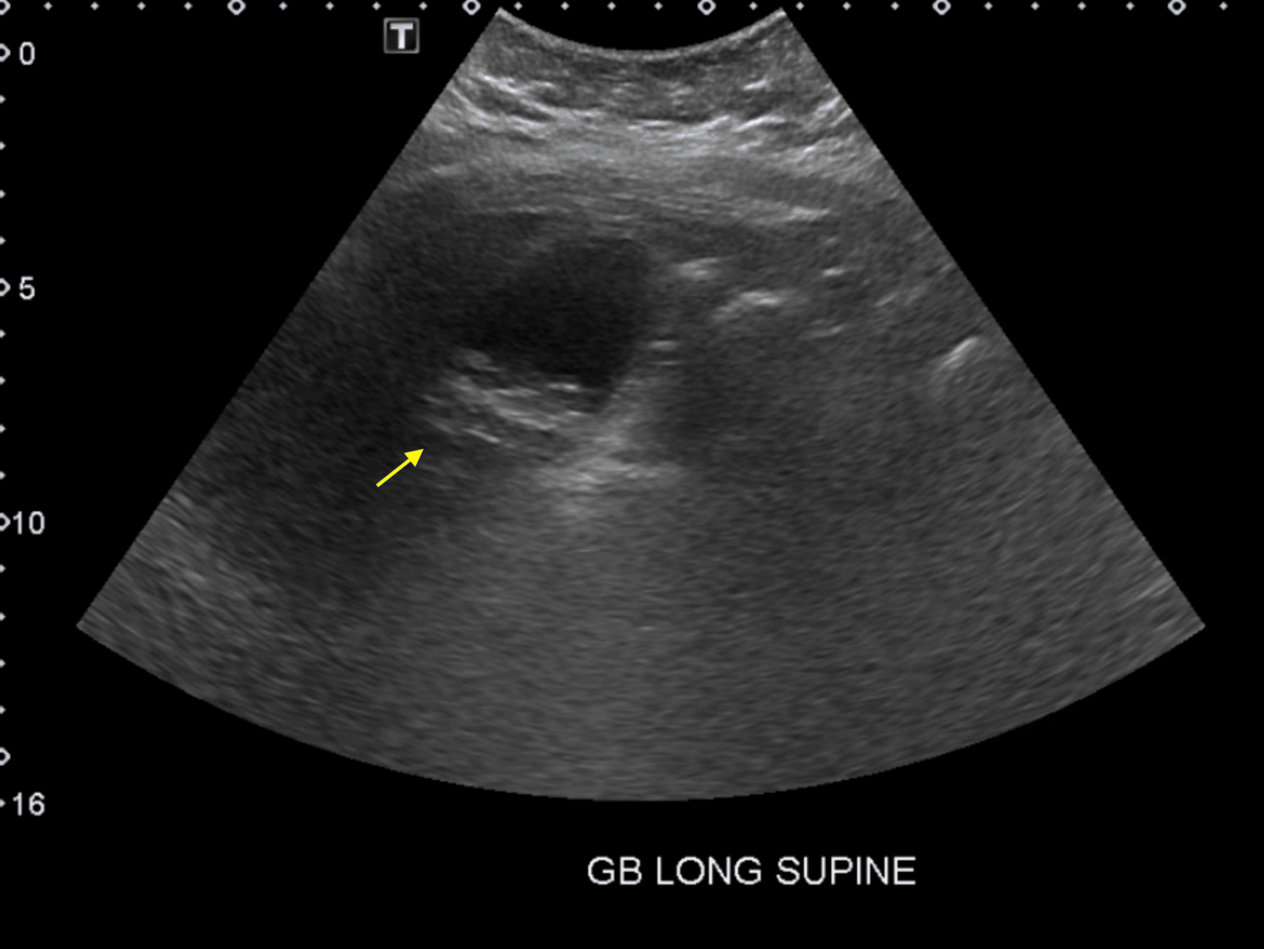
Gallbladder ultrasound in a long supine position Multiple echogenic, shadowing gallstones within gallbladder lumen; no pericholecystic fluid or gallbladder wall thickening
